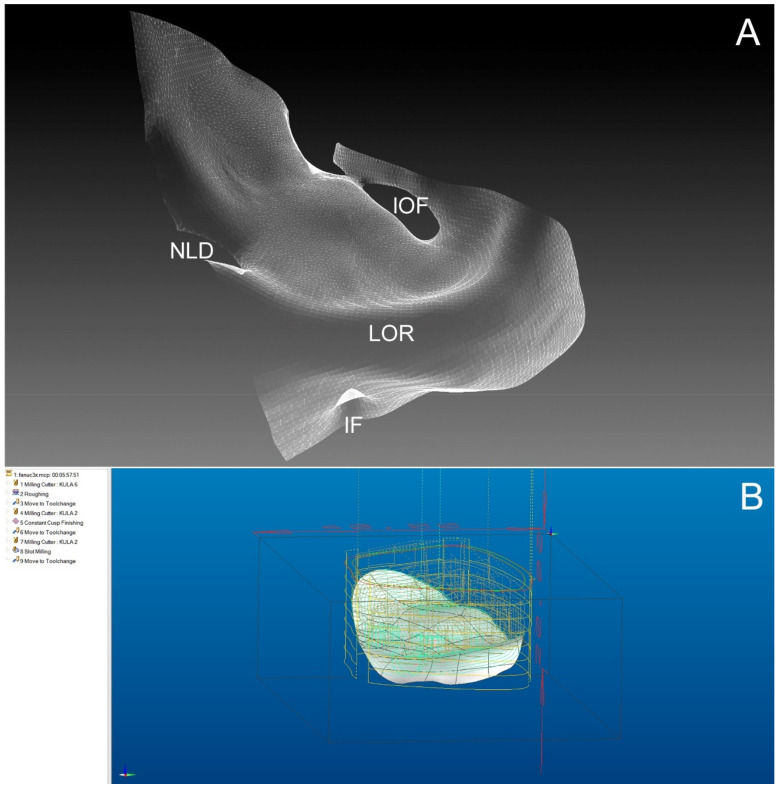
Segmented lower and medial orbital wall as shown by computed tomography. (A)—Creation of an intact orbit (IF—infraorbital foramen; IOF—inferior orbital fissure; LOR—lower orbital rim; NLD—naso-lacrimal duct). (B)—computer-assisted design (CAD). Design of custom implant-planning of the milling strategy for the zirconium oxide block. A 20% higher volume of implant is introduced.
